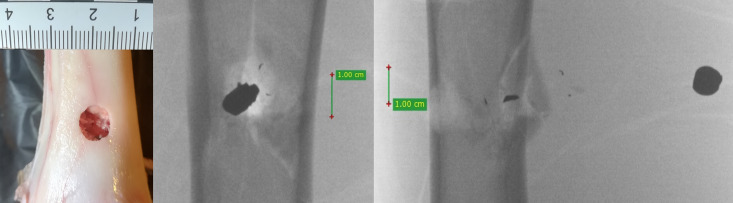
H&N Silverpoint airgun pellet cal. 6.35 mm, from the left: entry wound damage, A-P X-ray, lateral X-ray. Shot to the anterior surface of the femoral shaft.
